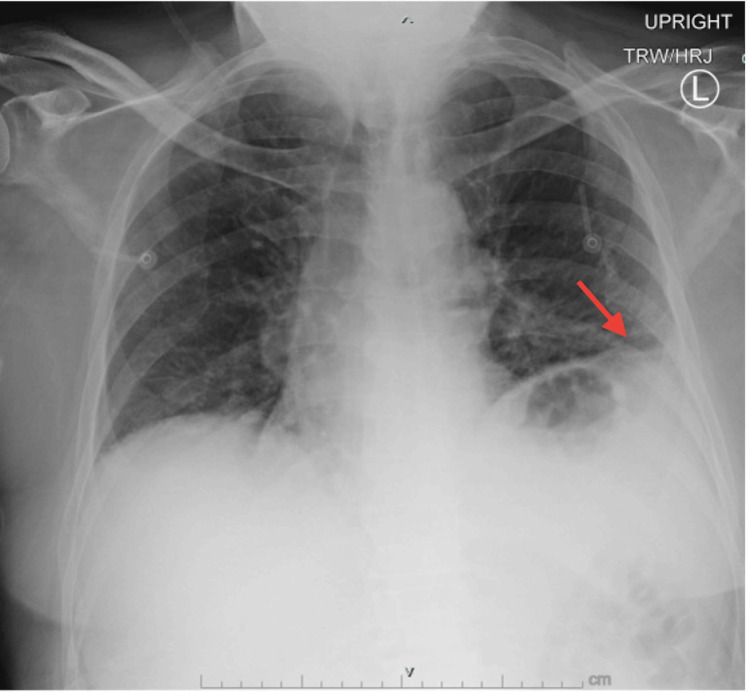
Chest radiograph showing low lung volumes and left basilar streaky opacity (red arrow) favoring atelectasis. No pleural effusions or pneumothorax are seen.
